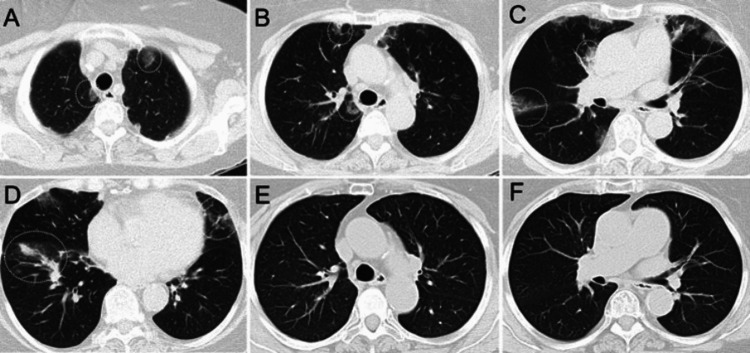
Chronic eosinophilic pneumonia in a patient with a history of asthma and peripheral eosinophilia. Axial CT images (A-D) show bilateral, multifocal, peripheral ground-glass opacities (some areas annotated with dotted circles) associated with reticular opacities and small consolidations. Axial images from a follow-up CT (E and F), after treatment with steroids, demonstrate interval resolution of lung opacities.
